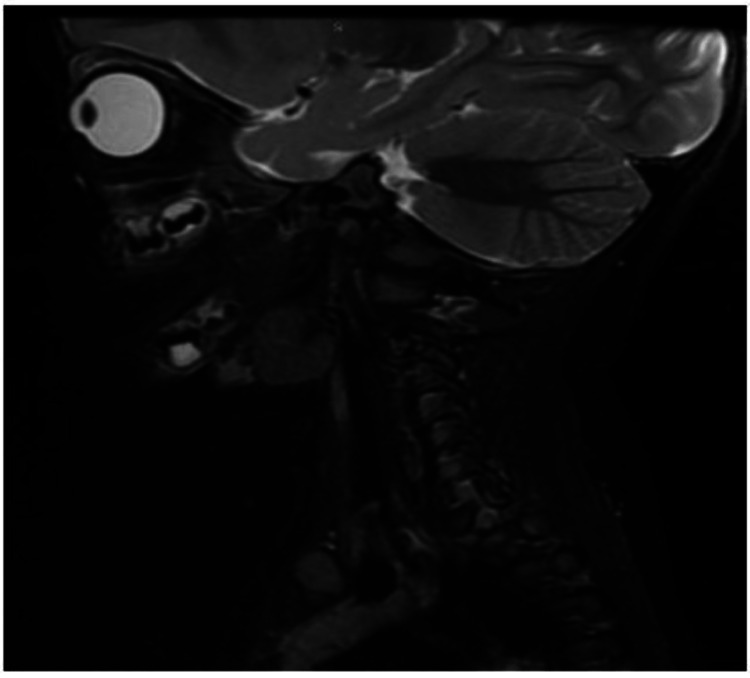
Sagittal view MRI of the neck The image shows complete resolution of the abscess with no evidence of collections or pathological lymph nodes and no anatomical defects.
